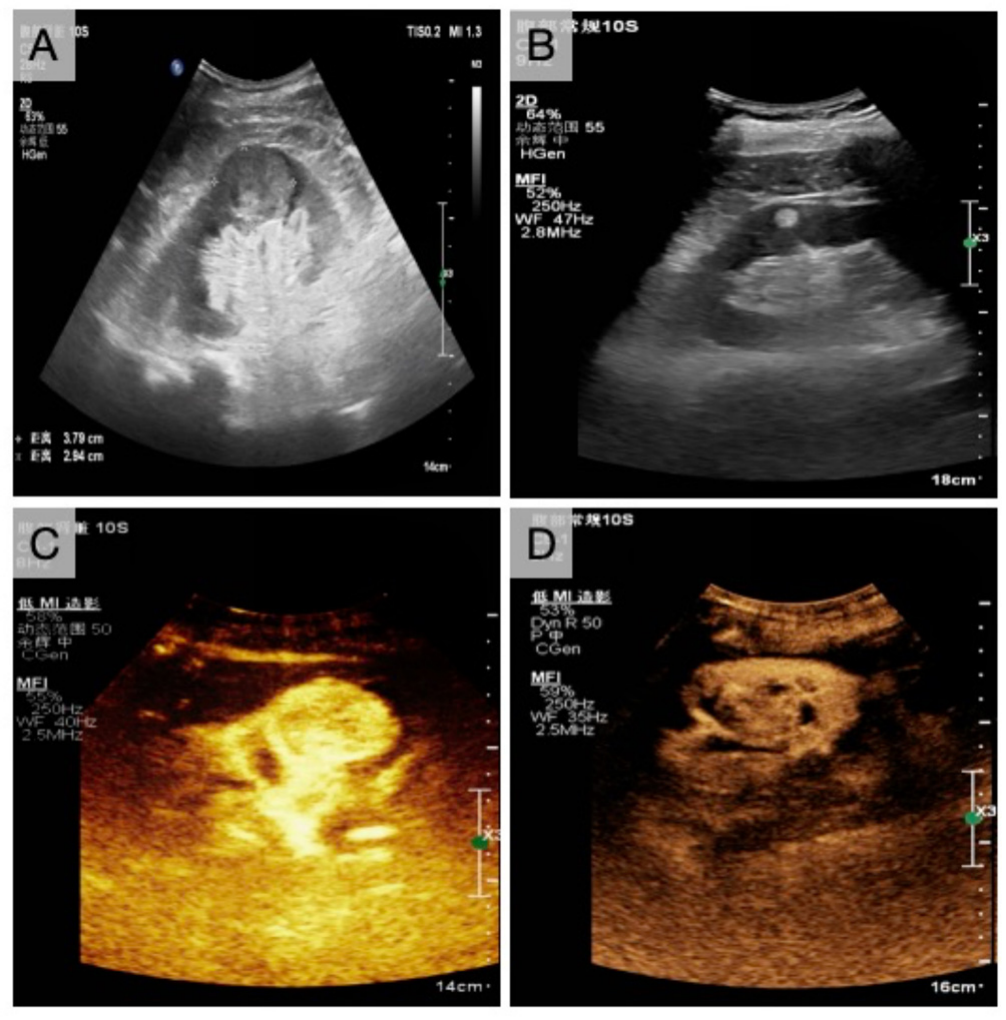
Images of benign and malignant lesions in renal tumors by CUS and CEUS. (A) Malignant and (B) benign tumors observed by CUS; (C) Malignant and (D) benign tumors observed by CEUS. CEUS: Contrast-enhanced ultrasound; CUS: Conventional ultrasound.
